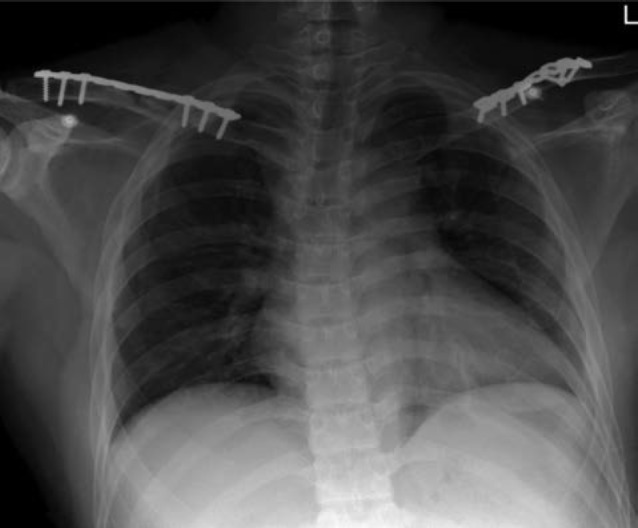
Radiographs immediate post-operation showed 3.5mm reconstruction plate used to fix the fractures.
